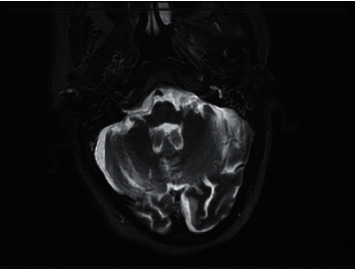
T2-weighted axial MRI following treatment: extensive signal changes in the left infratemporal fossa have resolved.
